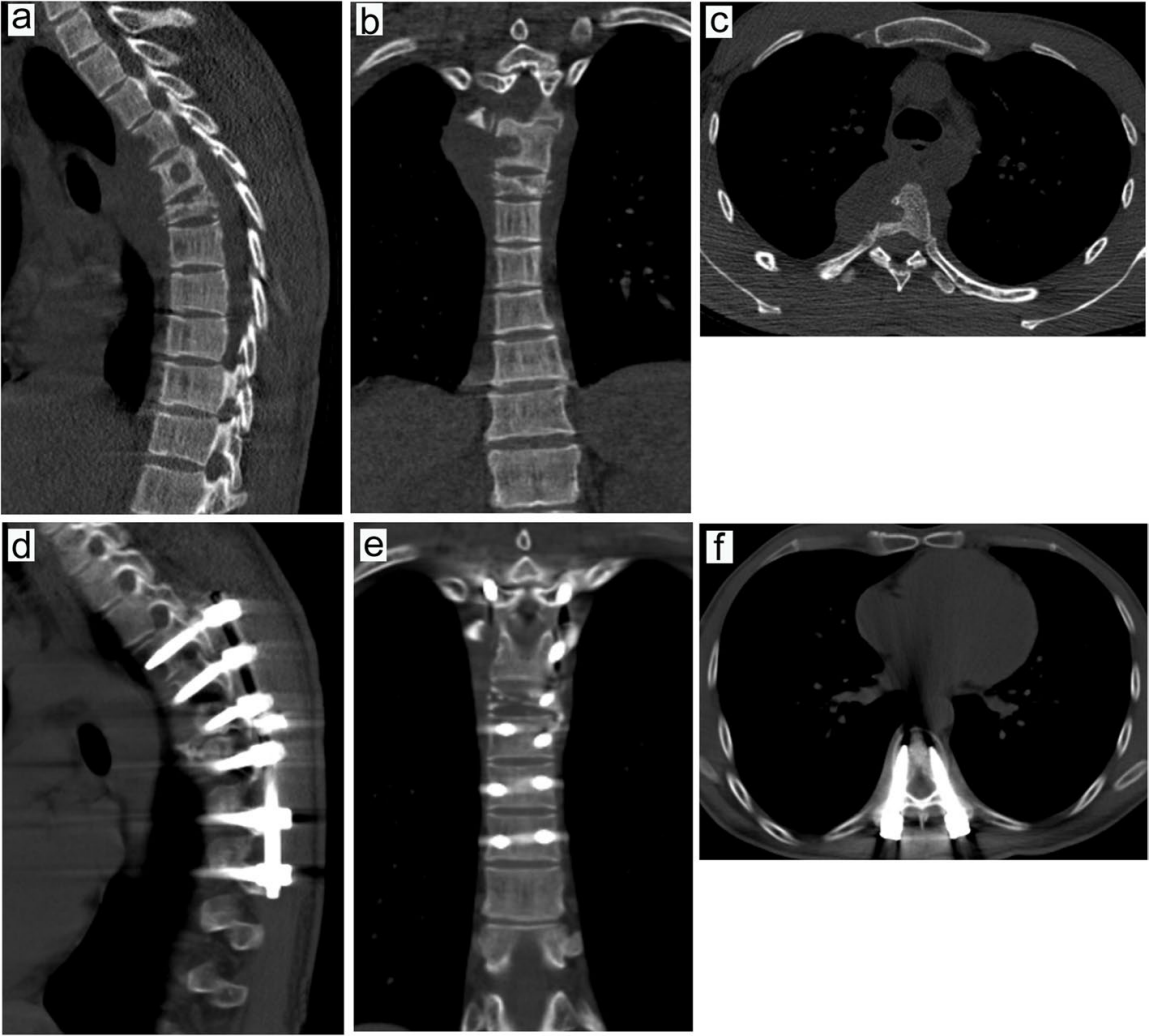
Preoperative and postoperative CT examinations in one patient without intraoperative blood transfusion. a, Preoperative CT examination in the sagittal position. b, Preoperative CT examination in the coronal position. c, Preoperative CT examination in cross-section. d, Postoperative CT examination in the sagittal position. e, Postoperative CT examination in the coronal position. f, Postoperative CT examination in cross-section
